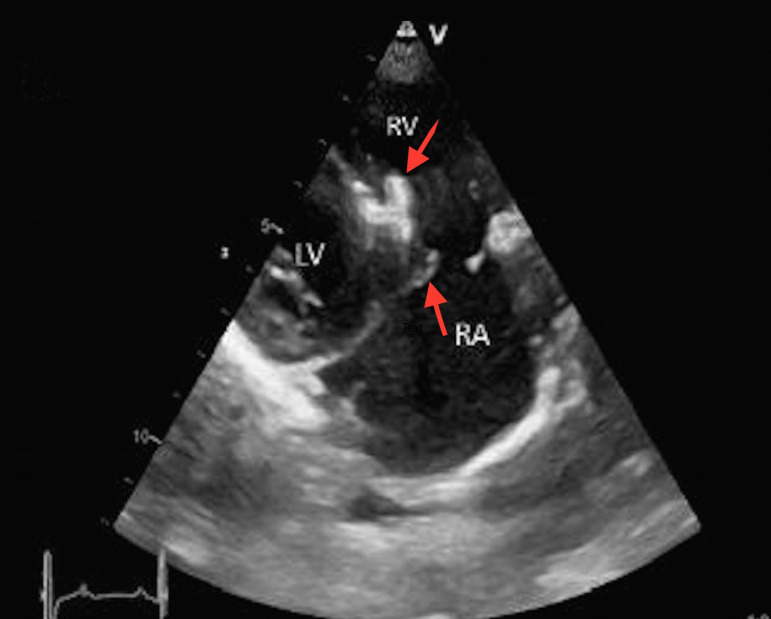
Transthoracic two-dimensional echocardiographic image of occluder device (upper arrow) and septal leaflet (lower arrow) entrapment during systole. LV=left ventricle; RA=right atrium; RV=right ventricle
